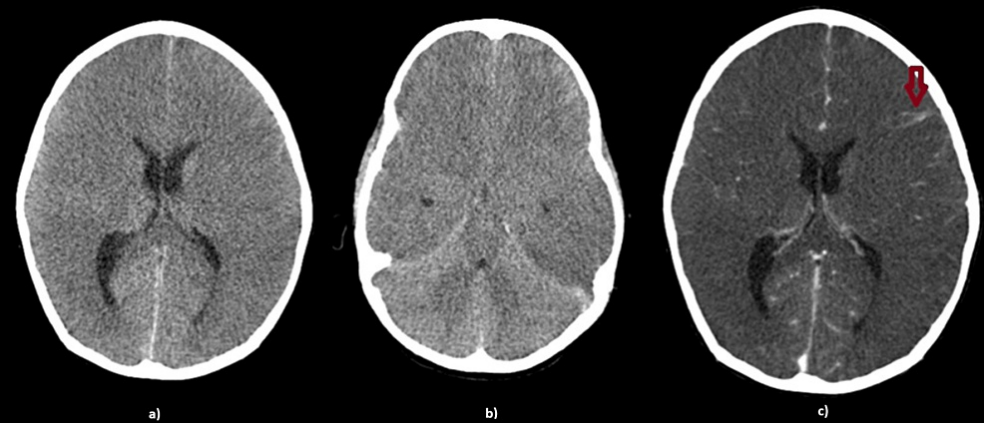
Head computed tomography scan showing a global effacement of the cerebrospinal fluid circulation spaces of the hemispheric sulci, convexity and basal cisterns, as well as a global loss of differentiation between gray and white matter in the supratentorial compartment (a and b). These findings are compatible with diffuse cerebral edema. After administration of contrast (red arrow on c), leptomeningeal contrast uptake is observed.
